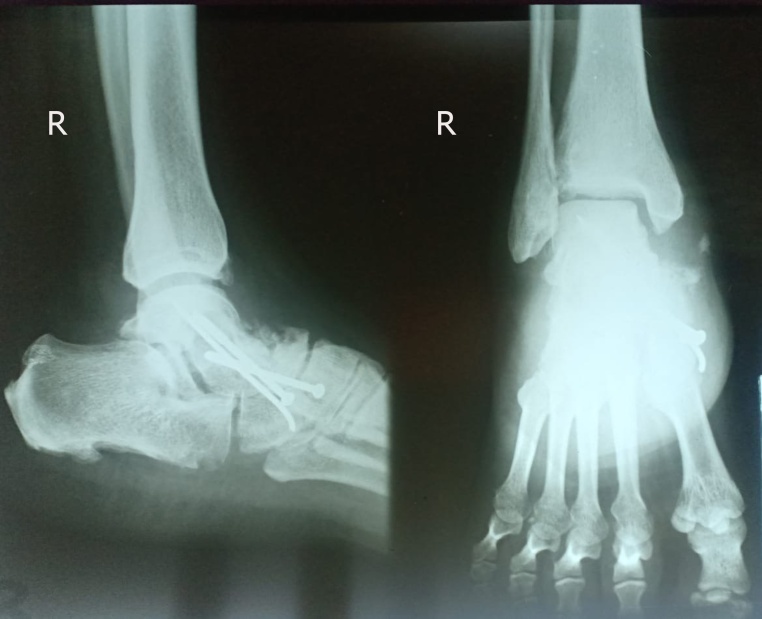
Plain radiograph in six months after forefoot arthrodesis showed the bony union and good improvement of Meary’s angle measurement at 8° from 22° before surgery.
